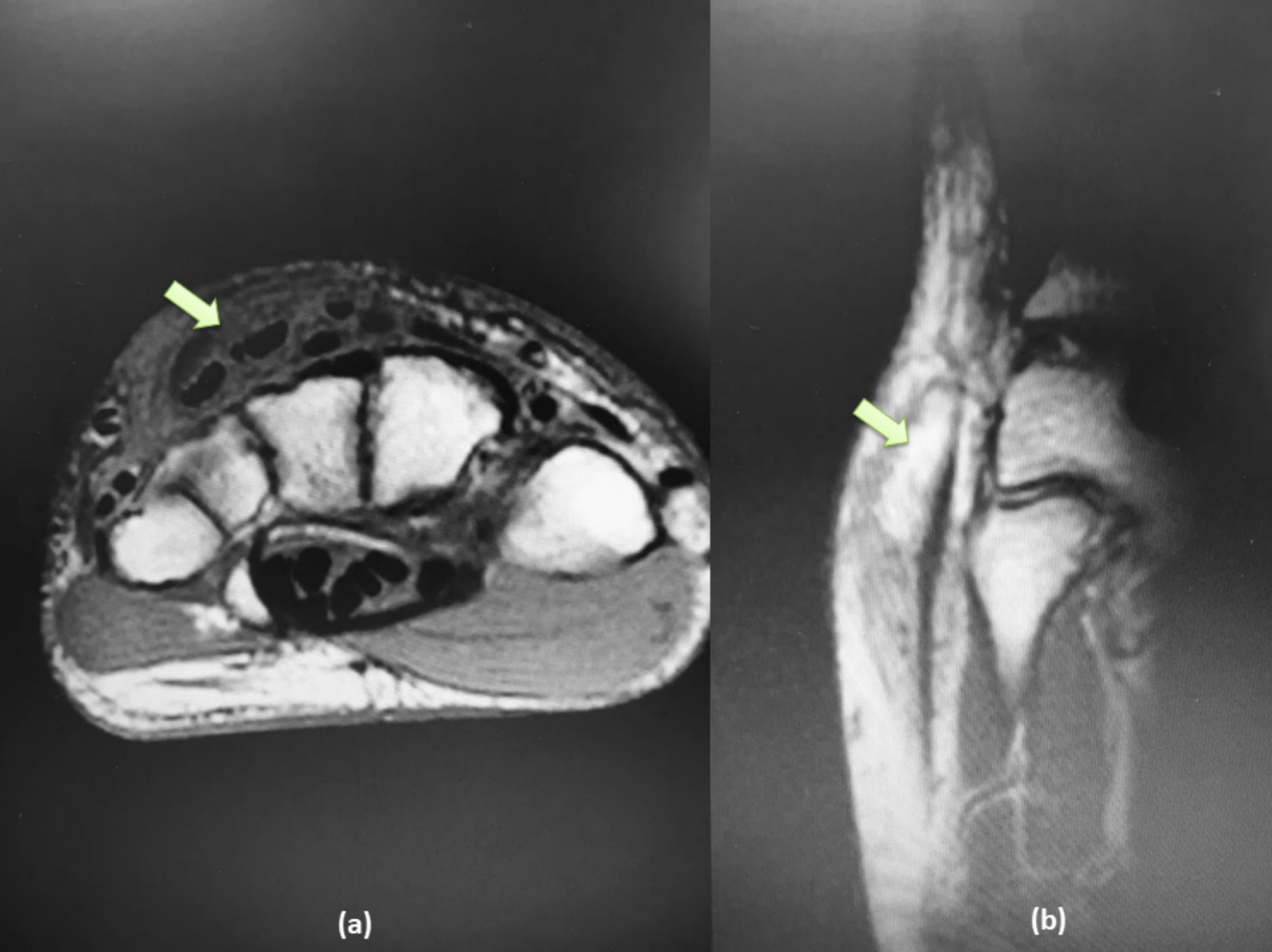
(a) Axial T1-weighted MRI cut showing well circumscribed mass circumferentially enclosing the extensors tendons of the hand, (b) sagittal T2-weighted images showing the extent of the mass
